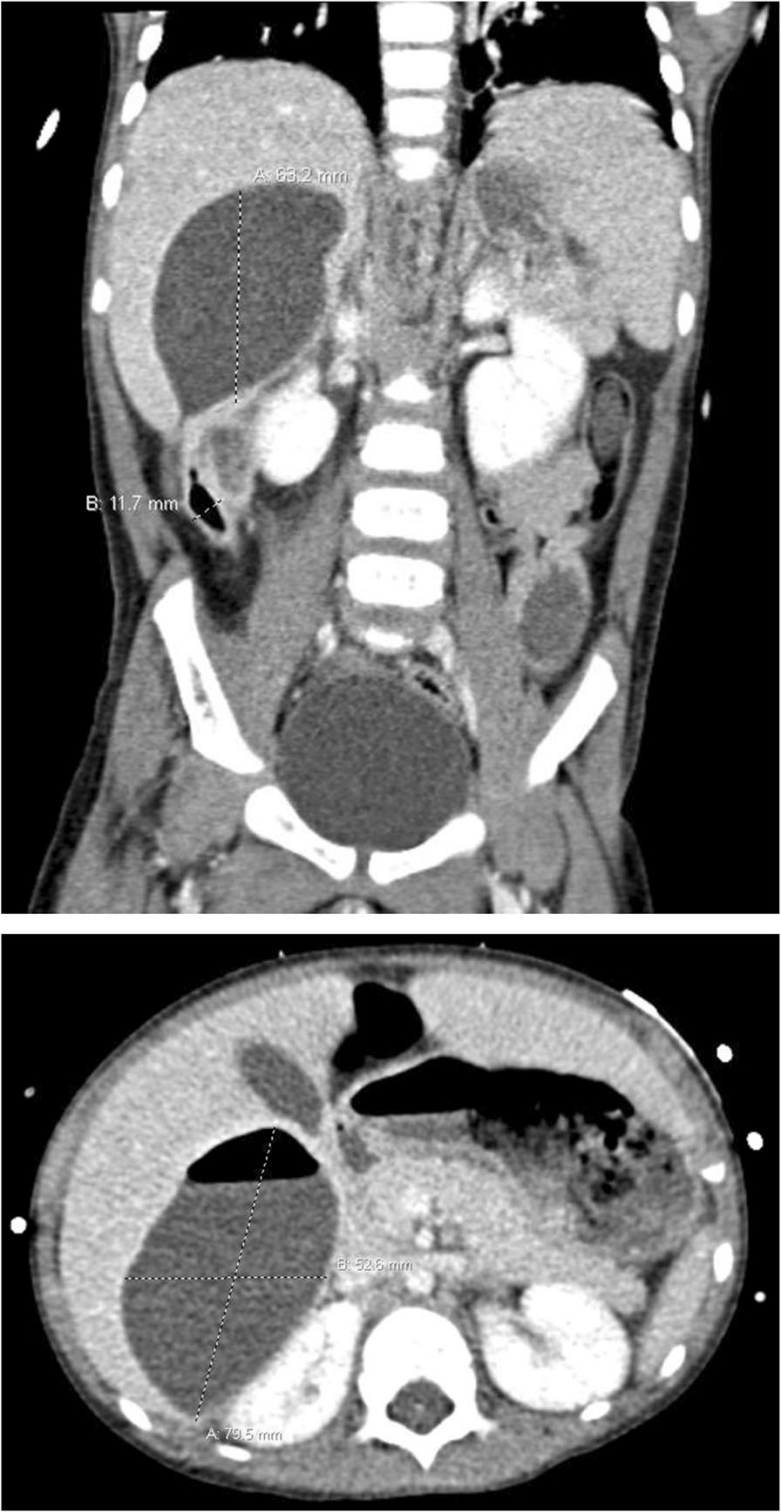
CT of the abdomen/pelvis with intravenous contrast revealing a retrocecal dilated appendix coursing cranially with adjacent inflammatory changes. Additionally, a large air-filled fluid collection is seen within the posterior right hepatic lobe measuring 9.0 × 5.3 × 6.3 cm. These findings are suspicious for an intrahepatic abscess
